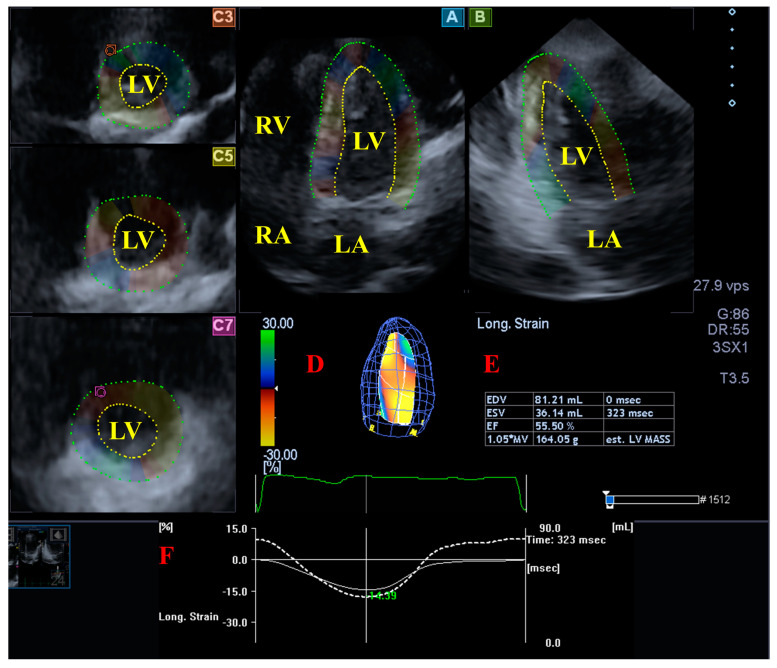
Analysis of the left ventricle (LV) from a three-dimensional (3D) speckle-tracking echocardiographic dataset: (A) apical four-chamber view, (B) apical two-chamber view and (C3) apical, (C5) midventricular and (C7) basal LV short-axis views. A virtual 3D cast of the LV (red D), LV volumetric data during the cardiac cycle (red E), time—LV global longitudinal strain (GLS, white line) and time—LV volume change (dashed white line) curves during the cardiac cycle (red F) are presented in a healthy subject. Abbreviations. LV = left ventricle, LA = left atrium, RV = right ventricle, RA = right atrium.
